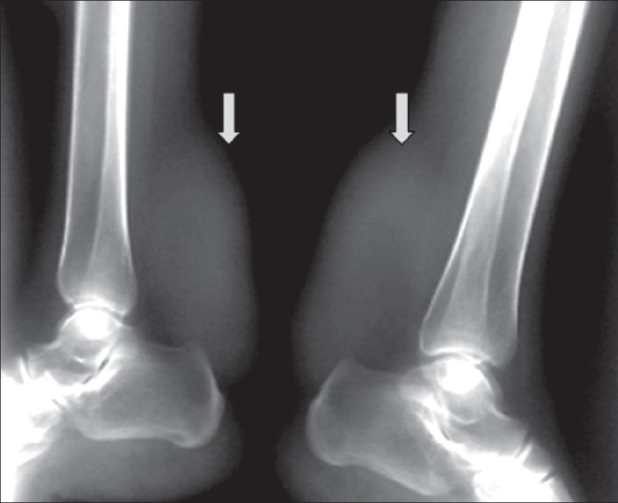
Plain lateral radiograph of both ankles showing bilateral symmetrical swellings (arrows) in the posterior aspect of the lower legs, superior to the calcaneum
